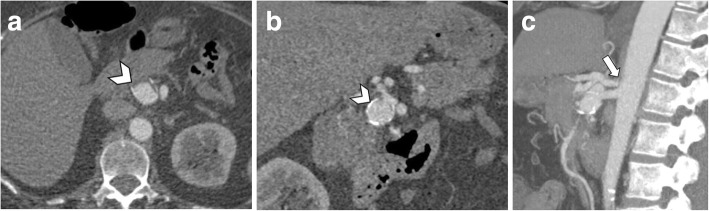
A 63-year-old woman with median arcuate ligament compressing the celiac artery. Axial (a) and coronal (b) arterial phase CT image shows pancreaticoduodenal artery aneurysm (arrowheads). Reconstructed MIP images on the sagittal plane (c) show severe stenosis at the origin of the celiac artery (arrow) with post-stenotic dilatation on arterial phase images acquired during expiration
